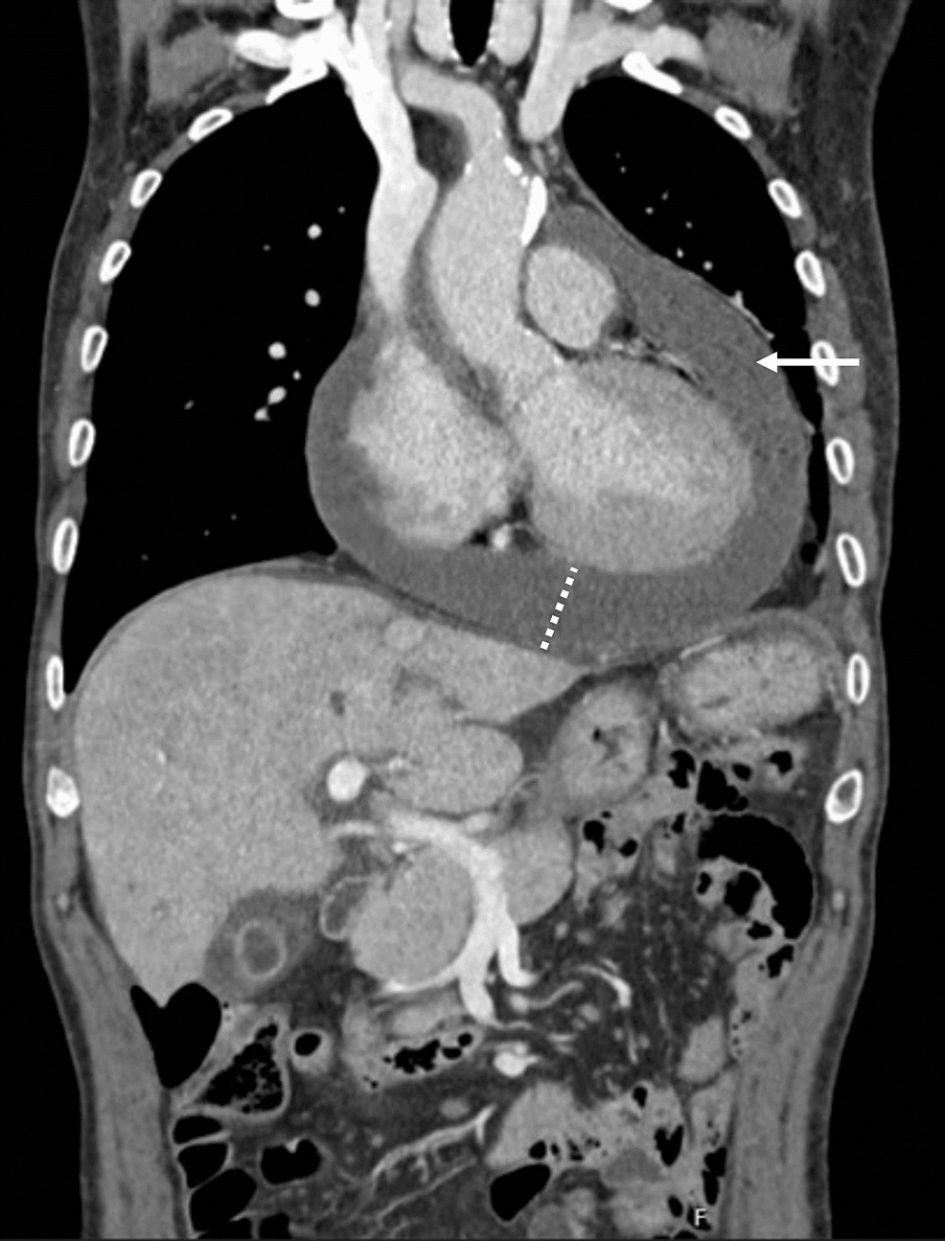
A coronal section of the thoracoabdominopelvic CT scan demonstrated pericardial enhancement compatible with active inflammation as well as a markedly large pericardial effusion.
